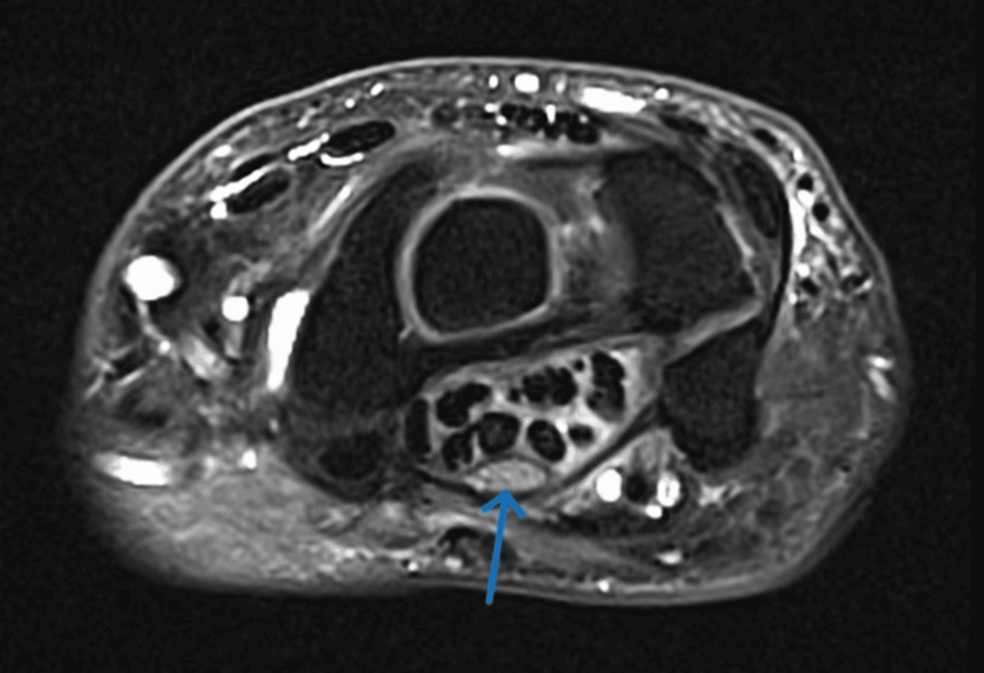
MRI showing median nerve thickening and edema within the carpal tunnel
